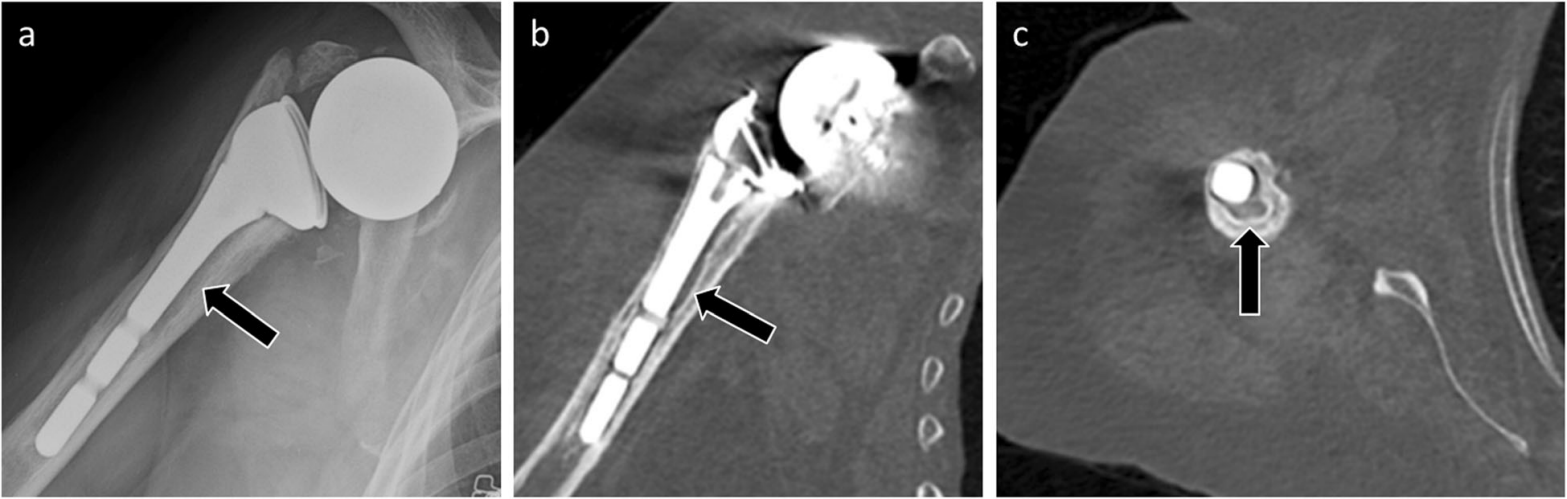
Anteroposterior radiograph (a) and coronal (b) and axial CT (c) images of a 79-year-old woman with humeral component loosening manifested by an irregular radiolucent line > 2 mm around the prosthetic stem (arrows)
